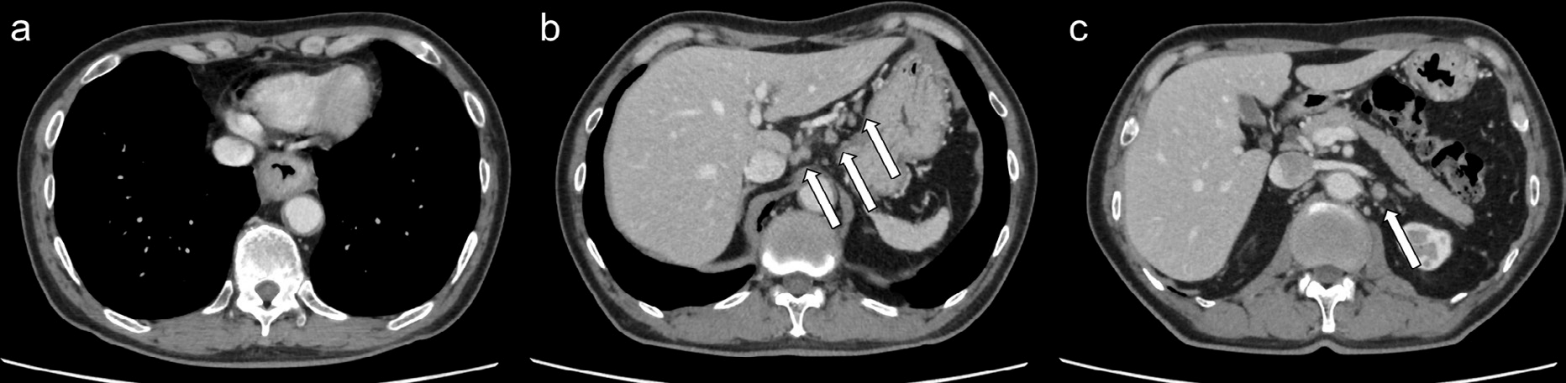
(a) Axial contrast-enhanced computed tomography shows the primary lower thoracic esophageal cancer. (b, c) Axial contrast-enhanced computed tomography shows lymph node metastasis involving the celiac, left gastric and para-aortic regions (arrows).
